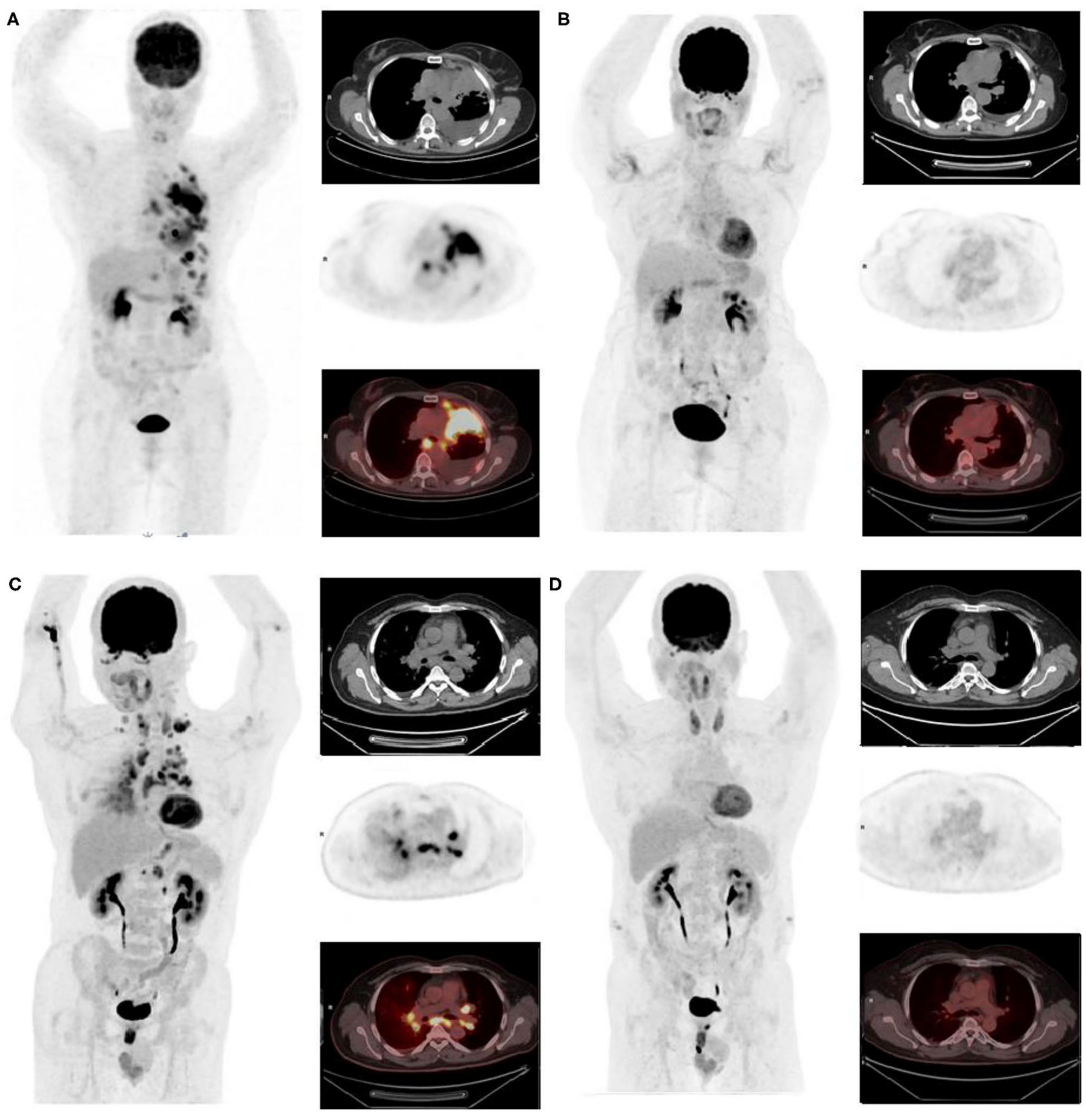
First, we report a non-smoker, 81-year-old African woman, diagnosed with ALK-rearranged lung adenocarcinoma on progressive dyspnea and fatigue with performance status (PS) = 2. A baseline ^18^F-FDG-PET/CT was performed, confirming a metabolic metastatic disease (left pleural nodes with pleurisy associated with upper and lower diaphragmatic lymph nodes) (A)
